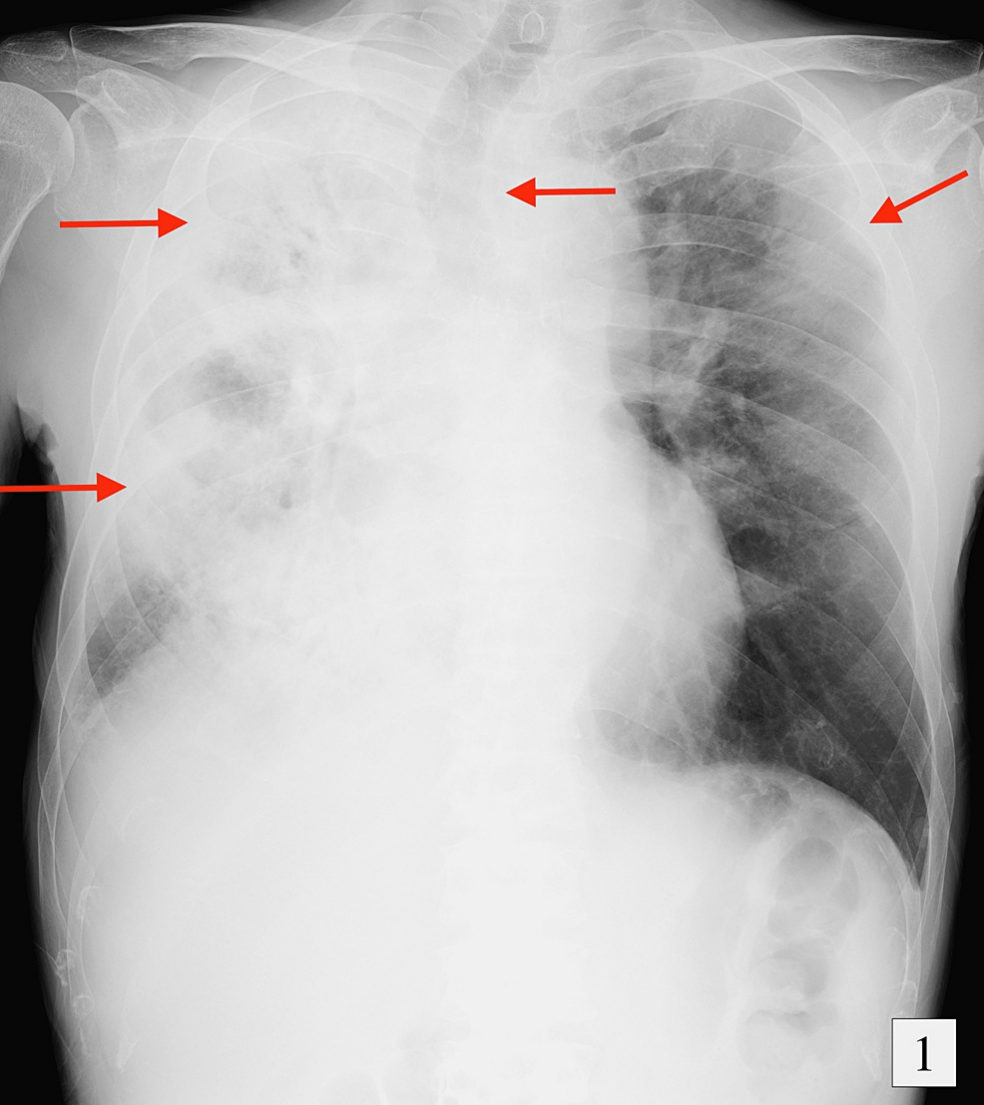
Chest radiograph on the first day of hospitalization showing tracheal excursion to the right side and consolidation in the right lung.
